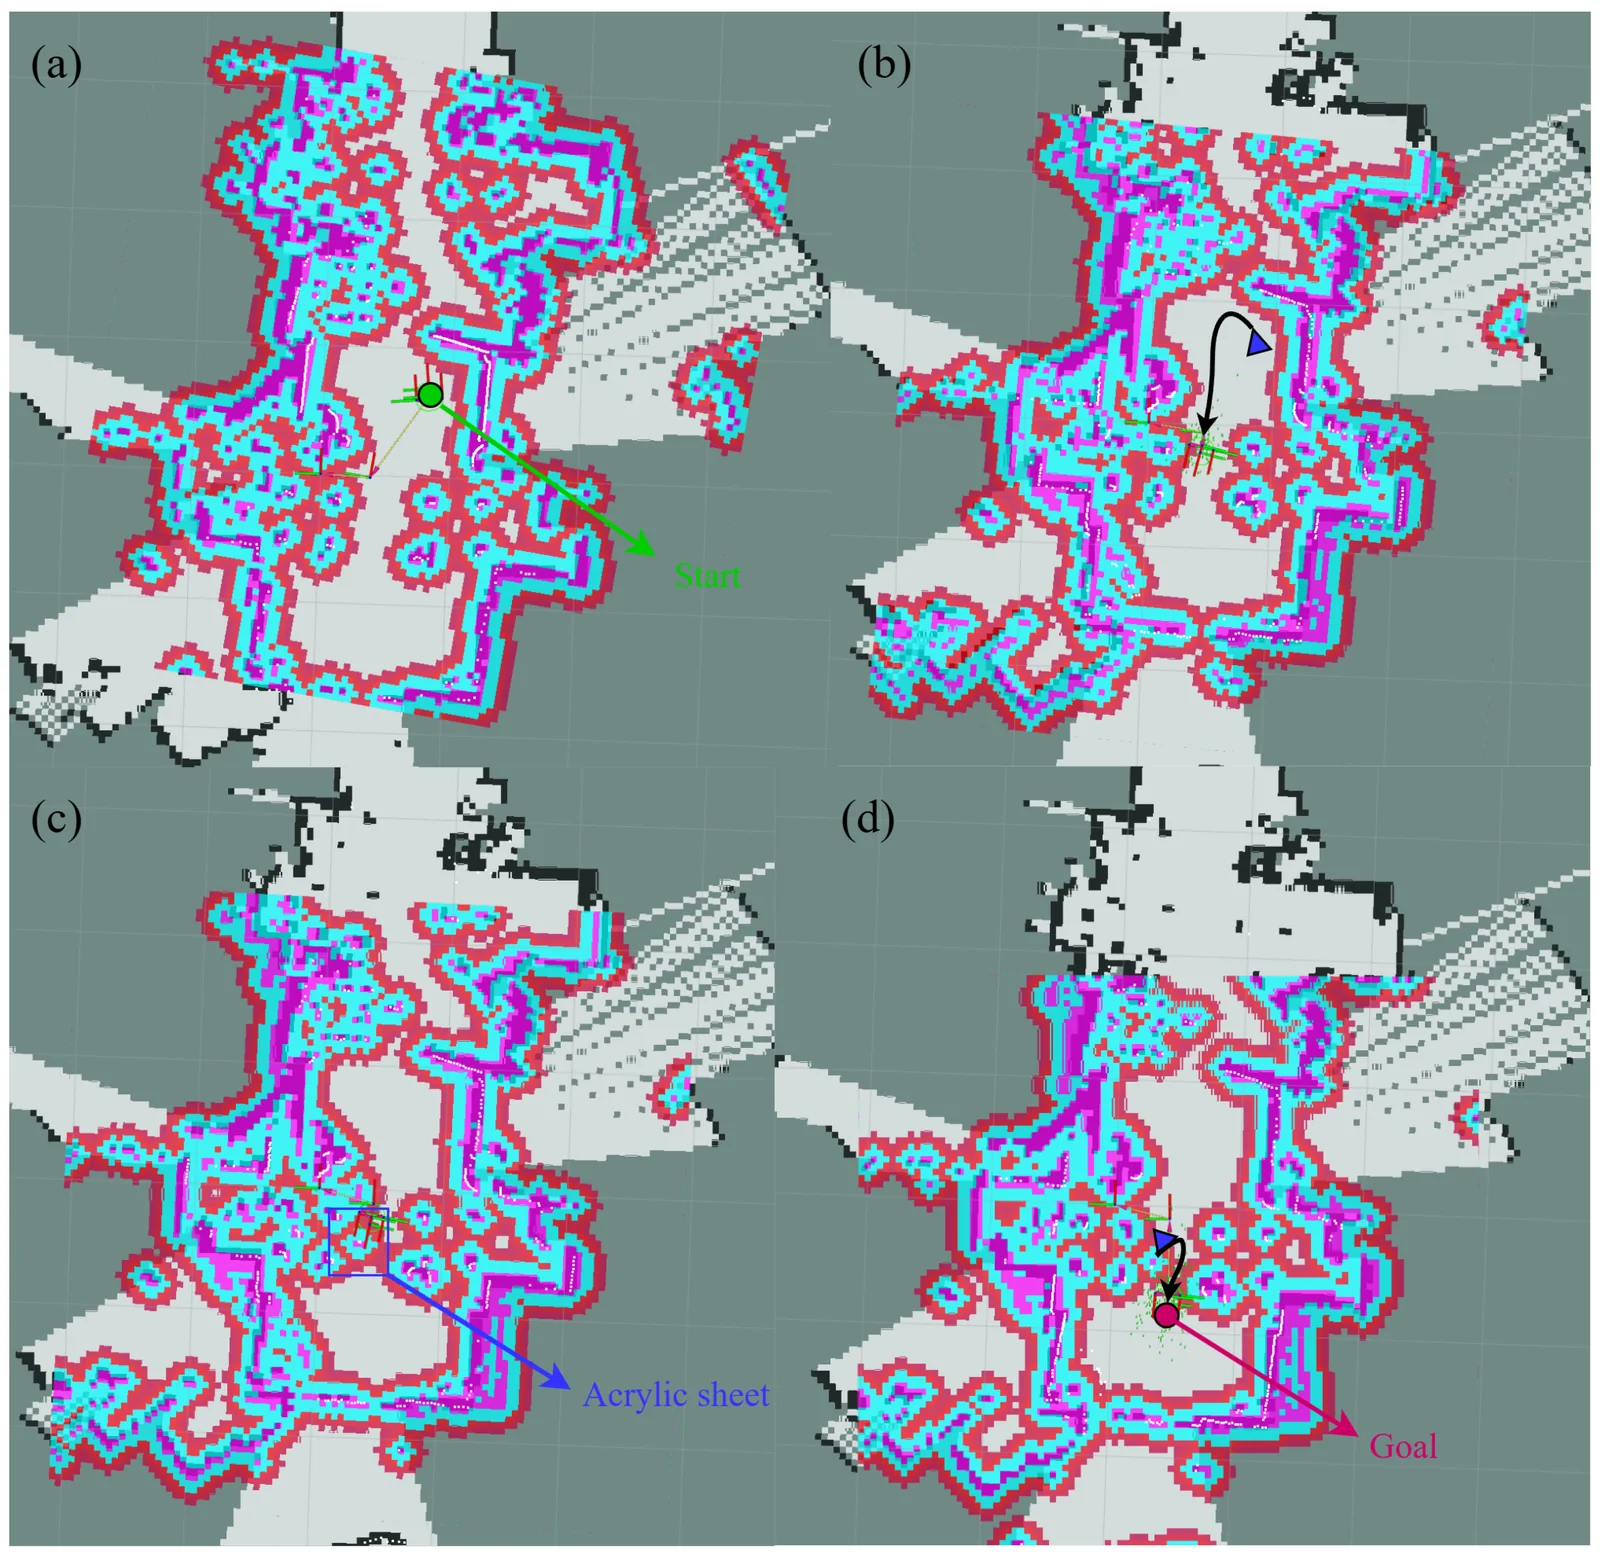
State sequence of transparent-obstacle avoidance in a narrow, obstacle-dense workshop-like layout. (a) Initial robot pose and goal command; (b) visual preemptive deceleration during the approach; (c) ultrasonic obstacle-point observation added to the local costmap near the acrylic sheet; (d) local-costmap update, local replanning, and goal-directed motion. Blue triangles denote the robot poses and headings, and black curves denote the representative travelled path.
